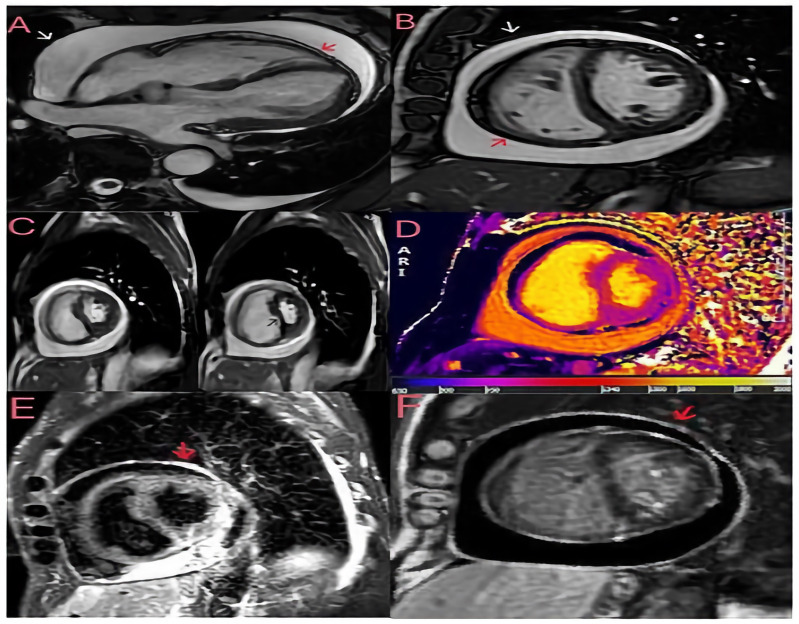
Cardiac magnetic resonance imaging (CMRI) was performed to assess the patient’s pericardial disease prior to referral to cardiac surgery. Breath-hold steady-state free precession (SSFP) cine of bright blood horizontal long axis (A) and midventricular short axis (B) views with evidence of a large pericardial effusion without compression of the ventricles in late diastole. The visceral (red arrow) and parietal (white arrow) pericardial layers are slightly thickened (3 mm). (C) Free-breathing SSFP cine in real time showing ventricular interdependence as a sign of constrictive pathophysiology; the left panel was taken during expiration, and the right panel during inspiration, showing septal bounce (black arrow) and crushing of the left ventricular chamber during inspiration. (D) The native T1 mapping sequence (modified look-locker inversion recovery) shows increased T1 times in the pericardium. (E) A short tau inversion recovery (STIR) T2 sequence showing pericardial hyperintensity (red arrow) compatible with oedema. (F) A late gadolinium enhancement (LGE) phase-sensitive inversion recovery (PSIR) sequence showing increased signal intensity in the pericardial layers (red arrow). The finding of a large pericardial effusion without tamponade and the widespread inflammation on the tissue characterisation images suggest subacute pericarditis with transient constrictive physiology, which accounts for 9–17% of cases of constrictive pericarditis and may resolve spontaneously within a few months [2,3]. Consequently, the patient was started on anti-inflammatory treatment with 600 mg of ibuprofen three times daily and 1 g of colchicine daily, resulting in clinical improvement, normalisation of inflammatory markers and discharge to home. During the follow-up, ibuprofen was progressively tapered to discontinuation, and at the last follow-up visit in October 2024, the patient was asymptomatic with no signs of volume overload and a normal plasma protein C level. This clinical case highlights the importance of assessing the chronicity of the disease by imaging in patients with constrictive pericarditis to differentiate between chronic and transient constriction, as acute/subacute cases may respond to medical therapy. Even though TTE is the imaging modality of choice for haemodynamic assessment, tissue characterisation with CMR is essential to diagnose the different phases of the pericardial inflammatory process, helping to differentiate an acute/subacute process (STIR+ and LGE+) from a chronic one (LGE+ and STIR-). Also of fundamental importance is the ability of cardiac magnetic resonance imaging to better assess the extent of pericardial effusion and pericardial thickening (a thickness > 3 mm tends to indicate chronic constrictive pericarditis). Such differentiation is crucial for tailored therapy; indeed, pericardiectomy is required in appropriate patients with chronic constrictive pericarditis [4]. In contrast, anti-inflammatory therapy is preferred in patients with transient constrictive pericarditis [5].
